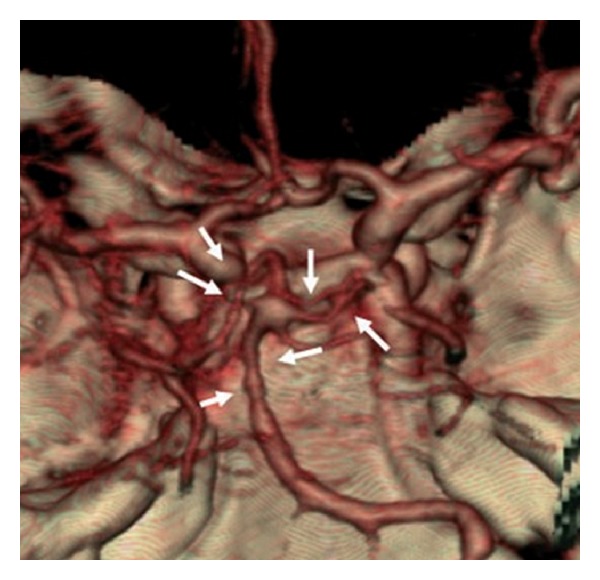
CT Angiography. Three-dimensional reconstruction shows severe vasospasm of the distal basilar artery and bilateral PCAs adapted from [1].
